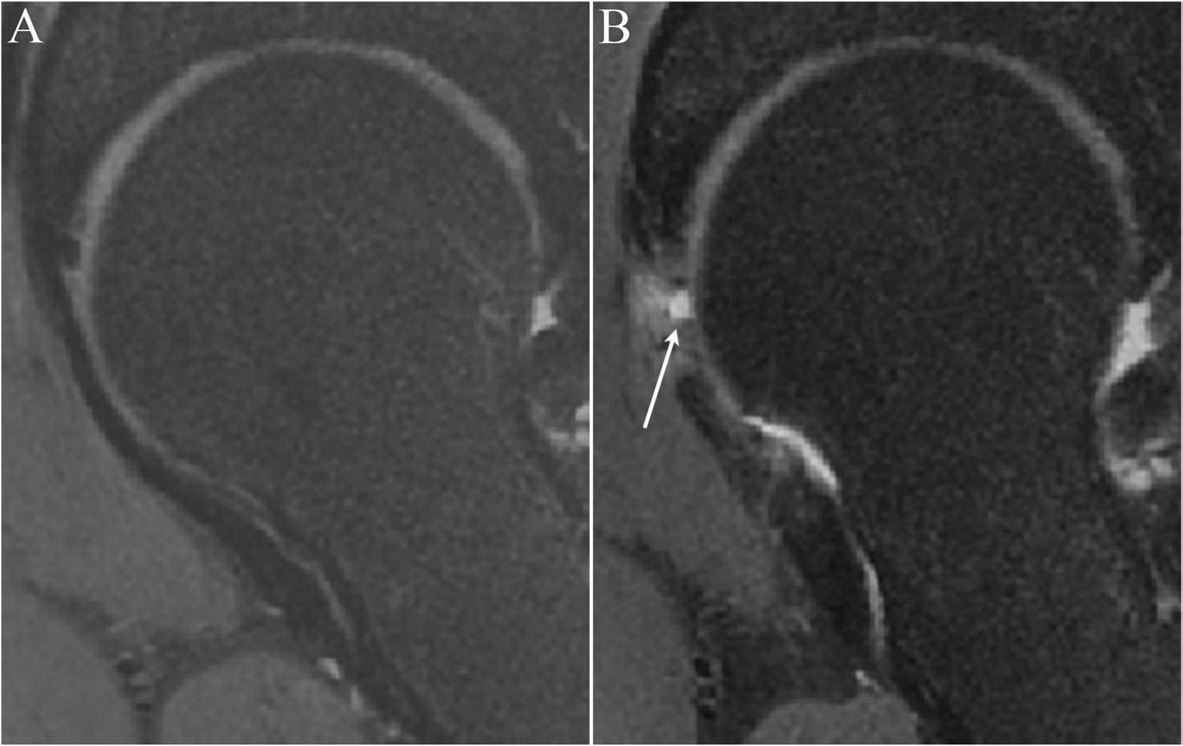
MRI measurement of pre- and postoperative anterior capsular thickness in one same patient. A, preoperative intact capsule; B, postoperative unhealed capsule (white line indicates the site of interportal capsulotomy)
